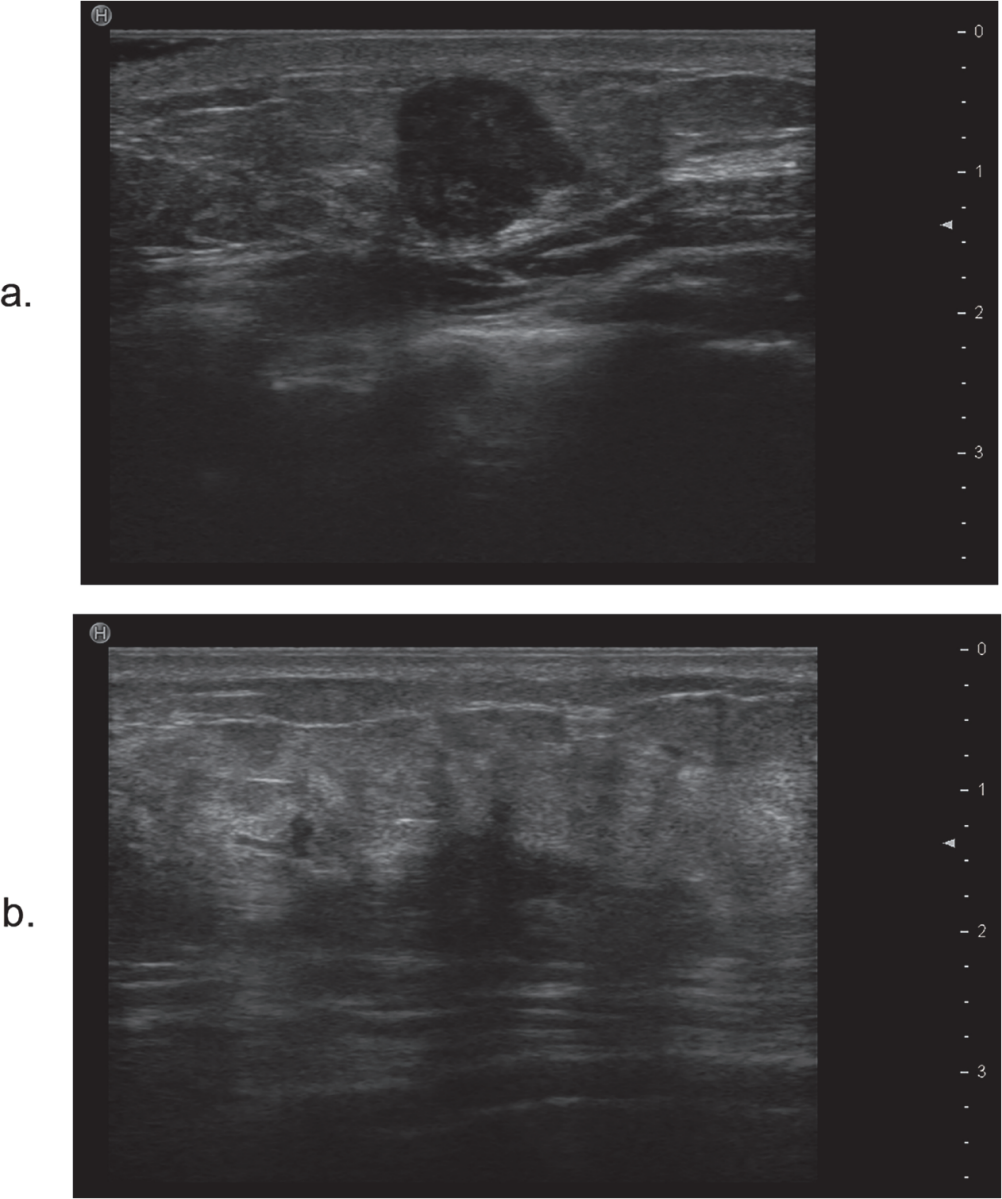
The tumor margin on ultrasound. a. It shows that there is a slight lobulated margin of normal-like breast tumor. b. The ultrasound image of a basal-like breast cancer shows that the tumor margin is angular and speculated.
